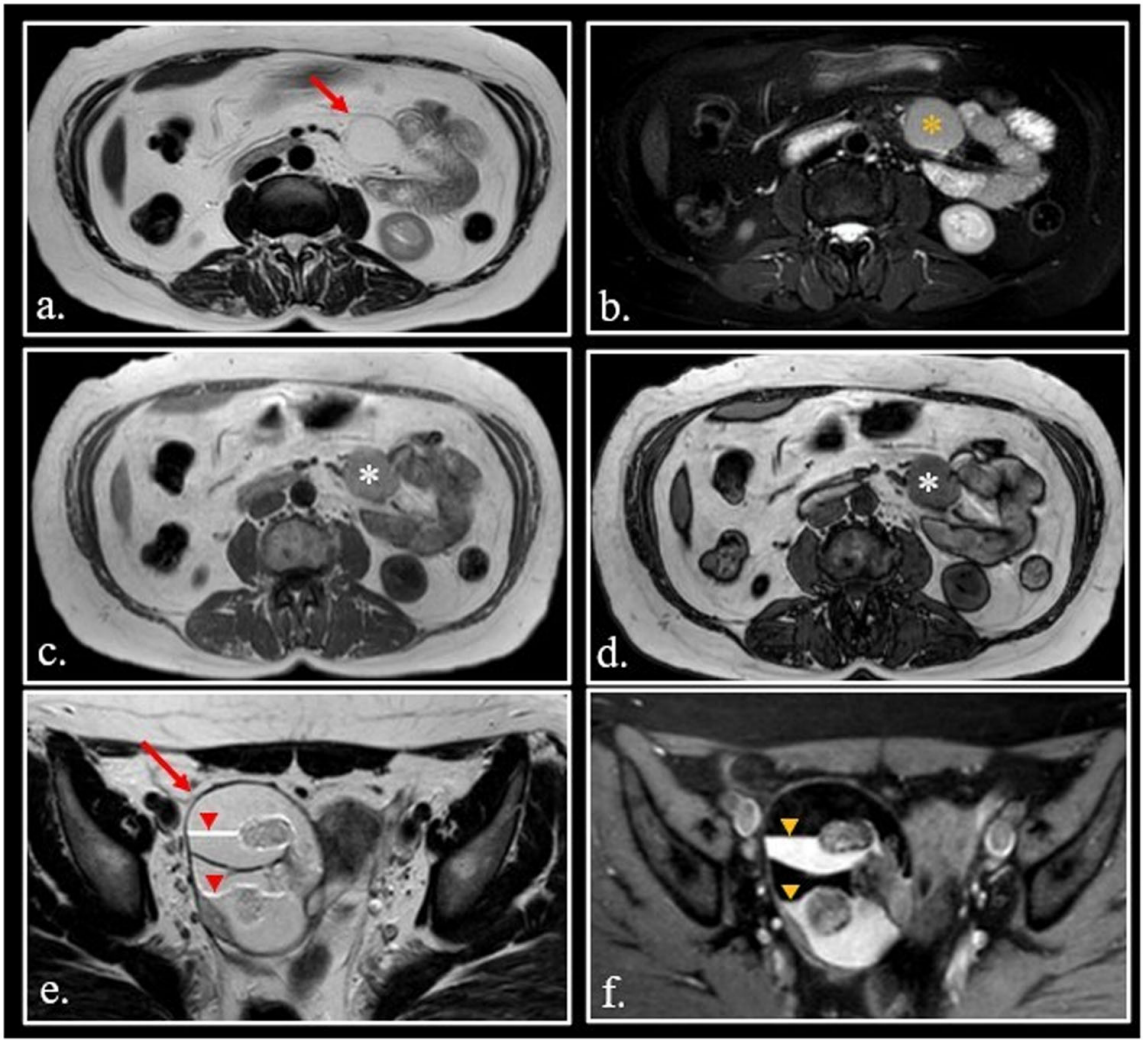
a–d Fast Spin-Echo (FSE) T2-weighted scan (a) of a 47-year-old female shows a cyst-like lesion of the mesentery root (arrow). FSE T2-weighted fat-sat (b) image demonstrates that lesion does not contain pure fat (orange asterisk). On T1-weighted in phase (c)—opposed phase (d) images loss of signal can be detected (white asterisk). Surgery confirmed the presumptive diagnosis of cystic lymphangioma containing chylous that is a mixed of water and triglycerides. e, f 25-year-old female with dermoid cyst (arrow). On FSE T2-weighted image (e), chemical shift artifact can be seen at the interface between upper fat layer and lower water layer with high-protein content (red arrowheads). On T2-weighted fat-sat (f), the chemical shift artifact disappears (orange arrowheads)
